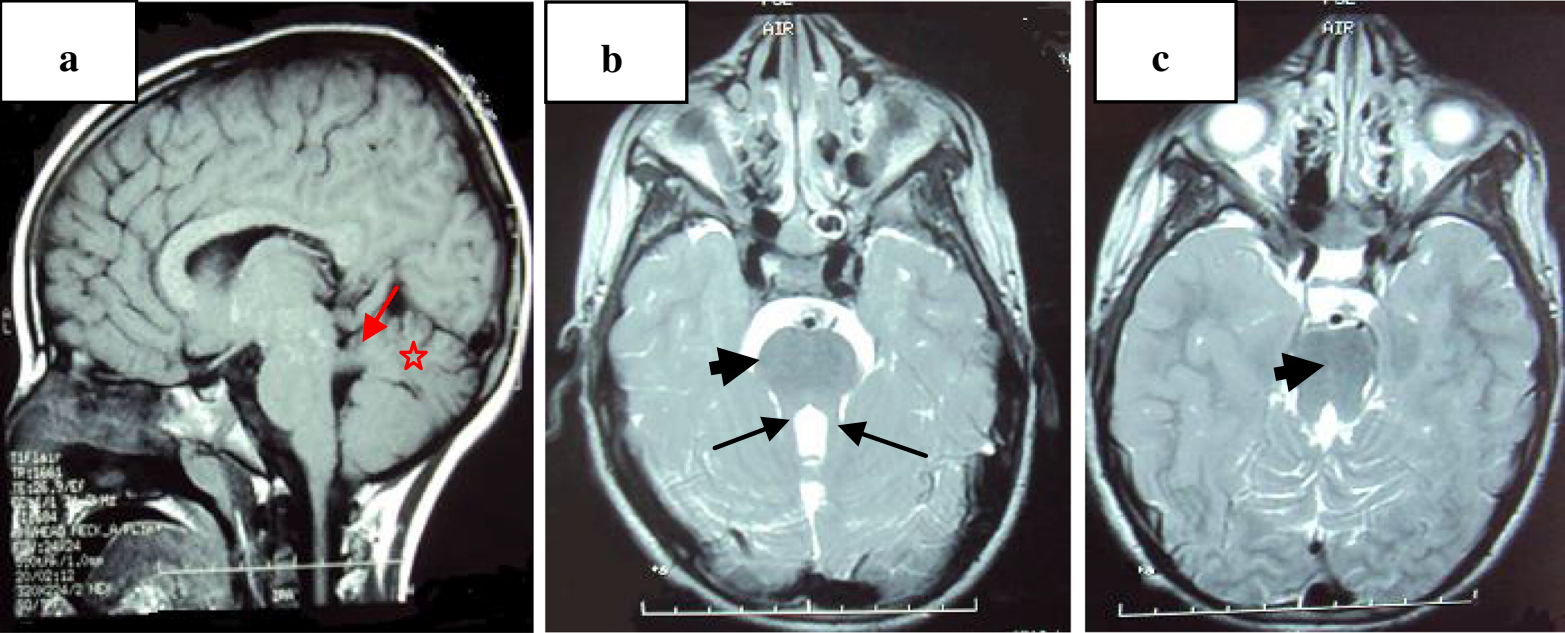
Magnetic Resonance Imaging (MRI): Sagittal and axial views through brainstem of patient 1 indicating hypoplasia of cerebellar vermis (red stars a), horizontally oriented (red arrow, a) and elongated (black arrows, b) superior cerebellar peduncles forming the “molar tooth“ sign (headarrows, b and c)
